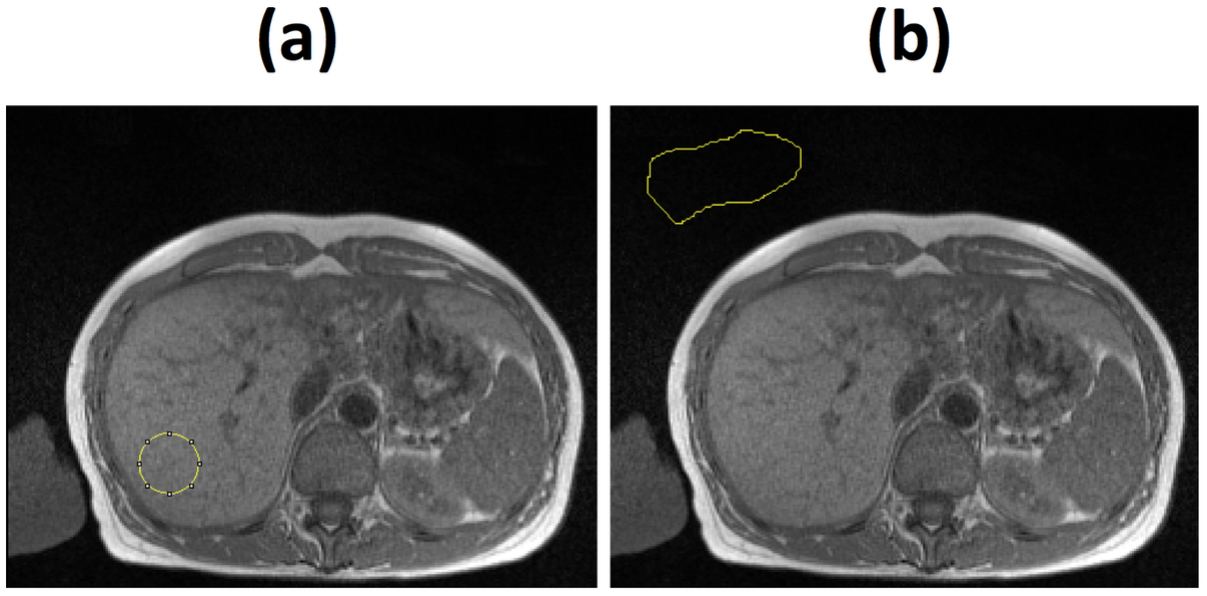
Magnetic resonance images of the liver. (a) A typical region of interest selected for analysis within the liver magnetic resonance image. (b) A typical region of interest of free space selected within the MR image for measurement of background noise.
